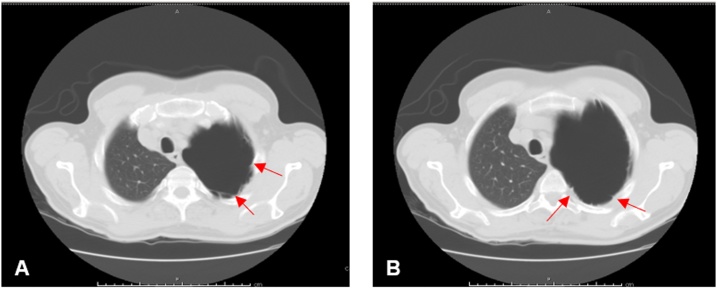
A–B. Dense pleural adherences visible on chest CT (arrows).
